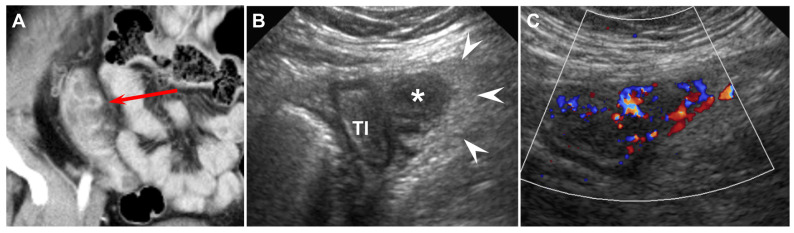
Forty-eight-year-old female (patient #8) with acute ileal diverticulitis. (A) Coronal reformatted enhanced CT scan reveals an outpouching diverticular sac (red arrow) connecting to the terminal ileum and mild ileal wall thickening. (B) US scan with C8-5 convex transducer demonstrates a homogeneous hypoechoic diverticular sac (*) connecting to the terminal ileum (TI), surrounding hyperechoic inflamed fat (arrowheads), and mild wall thickening of terminal ileum (TI). (C) Color Doppler imaging shows increased color flow to the diverticular wall and surrounding inflamed fat.
